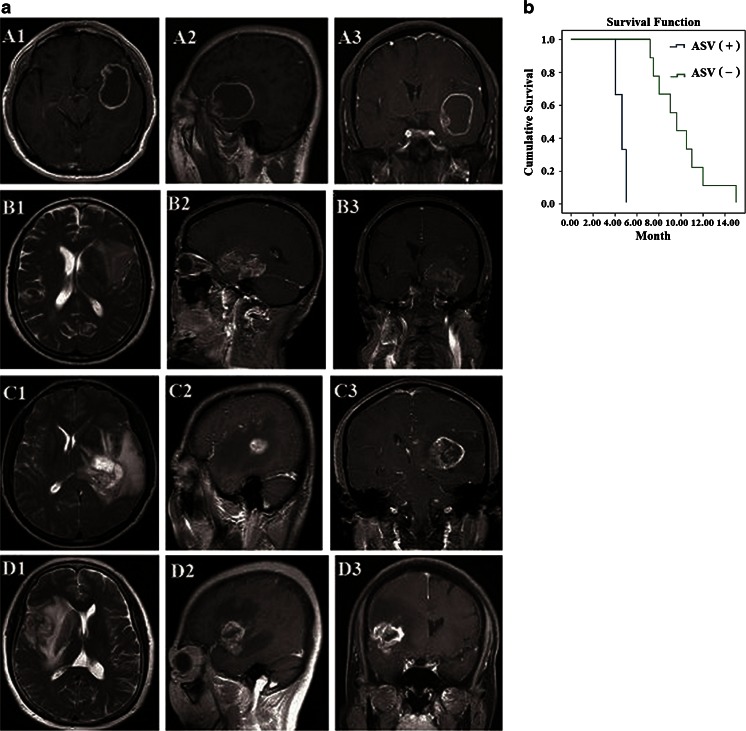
Magnetic resonance imaging (MRI) of glioblastomas and overall survival analysis of patients with GBM. a MRI of glioblastomas. *A* Patient with ADAR2 ASV– GBM. The lesion has invaded the temporal and frontal lobes. *B–D* Patients with ADAR2 ASV + GBM. *B* the lesion has invaded the temporal and frontal lobes. *C* The lesion has invaded the temporal, parietal, and occipital lobes. *D* The lesion has invaded the temporal, frontal, and parietal lobes. *A1* axial T1-weighted image; *A2, D2* sagittal T1-weighted images; *A3* coronal contrast-enhanced T1-weighted image; *B1*, *C1*, *D1* axial T2-weighted images; *B2*, *C2* sagittal contrast-enhanced T1-weighted image; *B3*, *C3*, *D3* coronal contrast-enhanced T1-weighted images. b Overall survival analysis of patients with GBM. The median survival time was significantly shorter in *ADAR2* ASV + patients compared with *ADAR2* ASV – patients (log-rank test, *p* < 0.0001)
